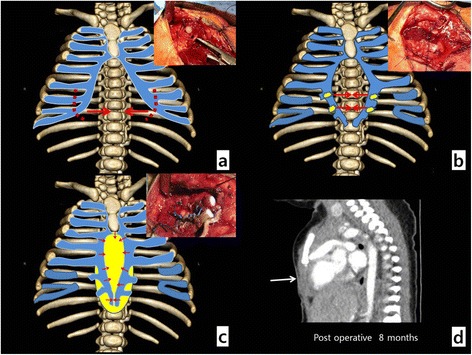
Operative findings. (a) Lowermost costal cartilage resection is performed for cartilage approximation; (b) Approximations of both costal cartilages are attempted, but the high tension of the string tying the cartilages prevents obtaining optimal results; (c) Permacol® patch of size similar to the defect is temporarily affixed onto the left costal cartilage by using a 3–0 Ethibond and cut into the appropriate size. The stitches on the right side is close to the left suture site to minimize the size of the Permacol® patch for the approximation of costal cartilages, and the bottom area of the patch is used to reinforce the diaphragm and peritoneum defect areas; (d) follow up CT shows no thoraco abdominal defect at the heart protruding site (white arrow).
